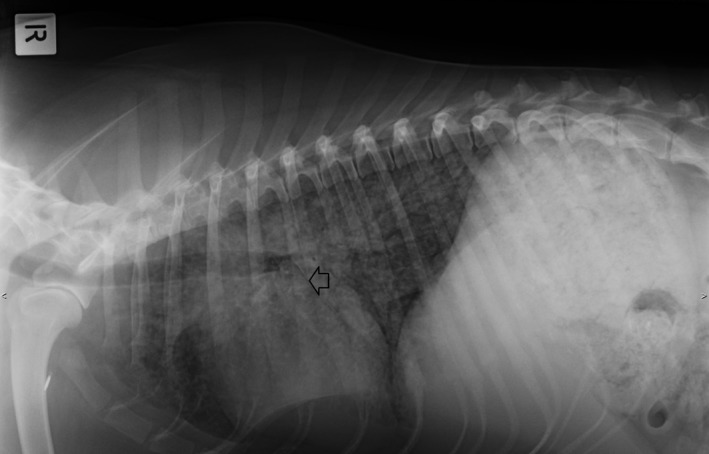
Right lateral thoracic radiograph obtained at Day 11. A diffuse, predominantly interstitial pattern is evident. Arrow shows fissure line
